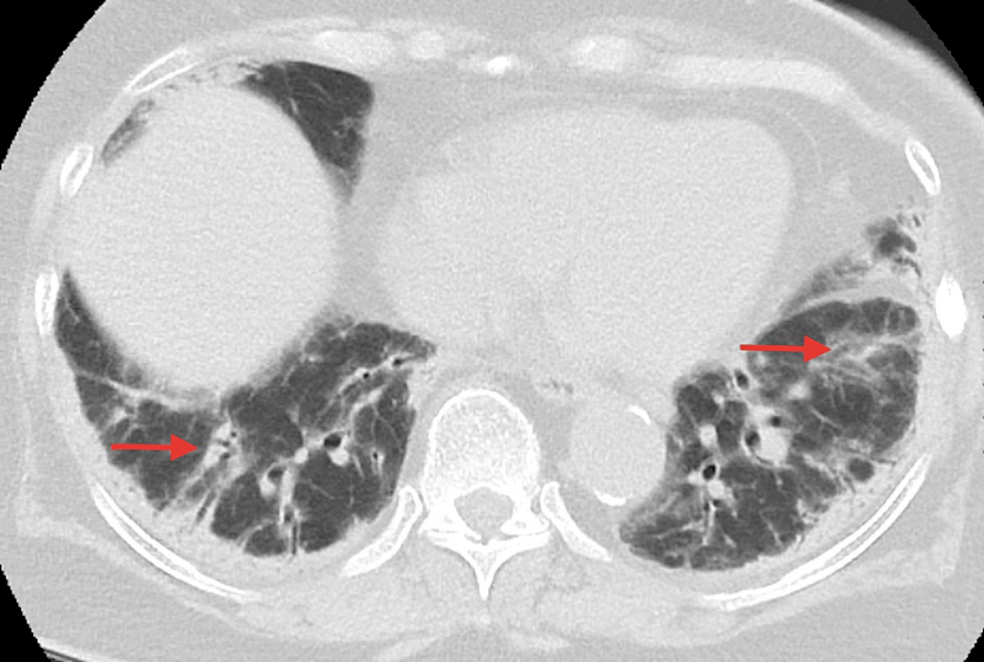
CT chest showing lung bases with bilateral pleural-based ground glass opacities
